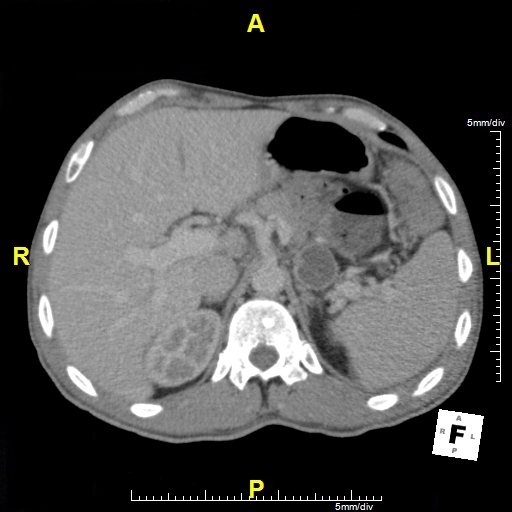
CT scan showing cystic lesion of the pancreas. Thin walled lesion without segmentation with homogenous fluid content
